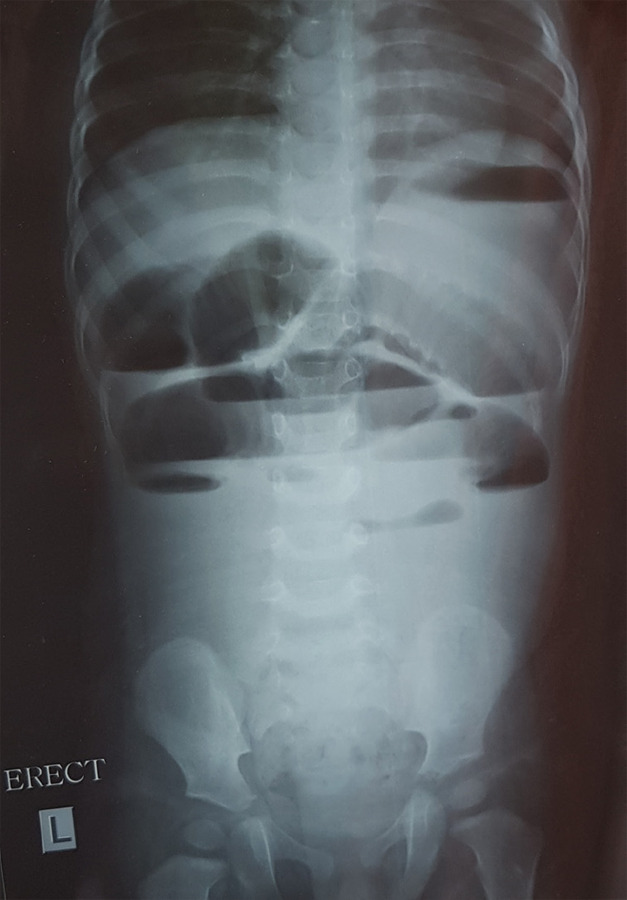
Plain radiograph of the abdomen showing multiple air-fluid levels.
